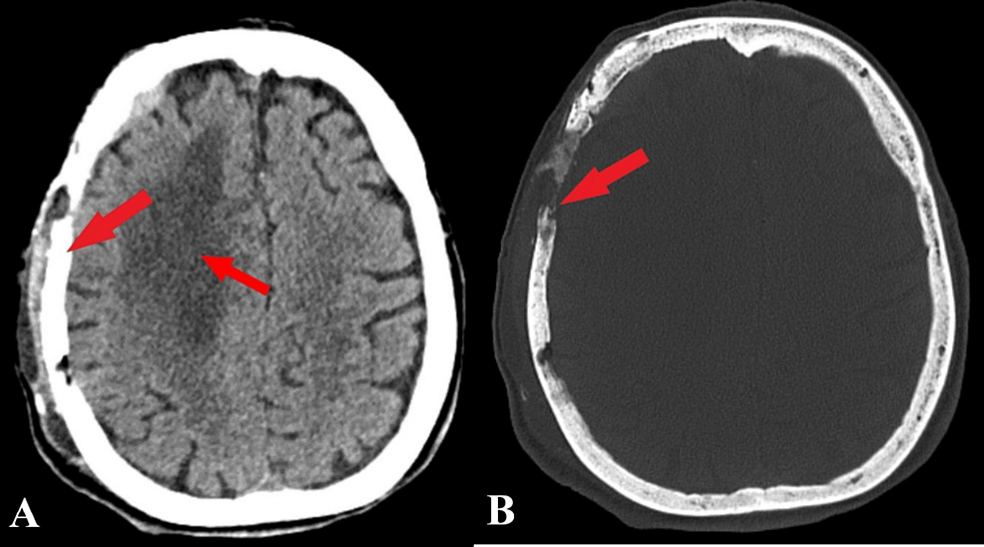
Postoperative CT CT tissue window (A); the red arrows indicate the gross total tumor resection, while the CT bone window (B) shows the postoperative still skull lysis (pointed by the red arrow) due to extracranial invasion.
